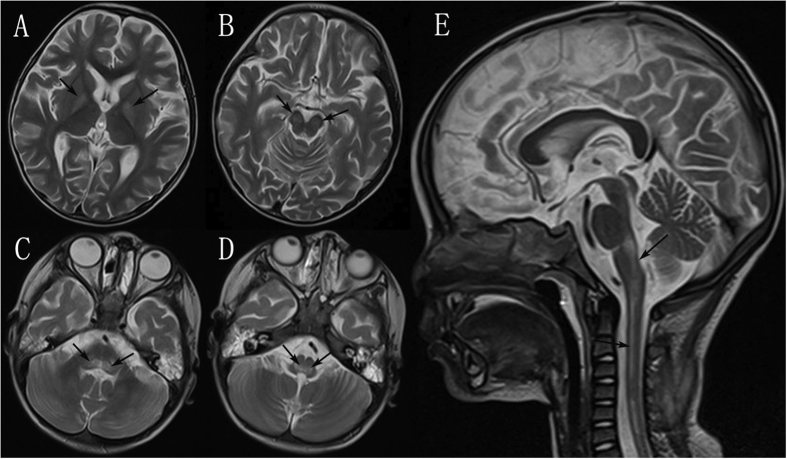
Spin-Echo T2-weighted MRI scan of a 28-month-old boy with encephalitis, brainstem encephalitis and myelitis from EV-A71 infection. The child had fever; rashes on the mouth, palms, and soles of the feet; vomiting; myoclonus; tachypnea; and drowsiness, followed by coma. Physical examination revealed skin rashes, weakness in four limbs, hypermyotonia, and positive reflex of Babinski’s sign. At the basal ganglia level (Panel A), there was an increased signal intensity of the bilateral globus pallidus (arrows). At the midbrain level (Panel B), there was an increased signal intensity of the bilateral cerebral peduncle (arrows). At the pons and medulla oblongata level (Panel C,D), lesions of high signal intensity were observed in the bilateral tegmentum (arrows). In the sagittal view (Panel E), there was a high signal intensity from the brain-stem to the anterior horn of the cervical spinal cord (arrows).
